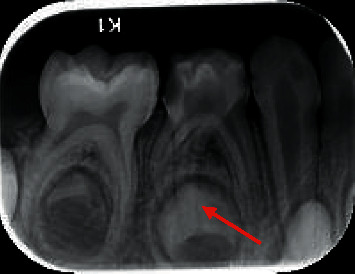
Intraoral periapical radiograph showing third root in right mandibular first primary molar.
